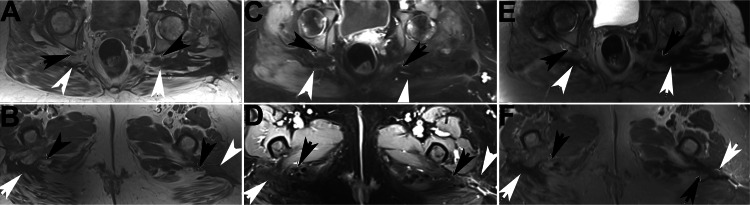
Sequential axial MR images of Case 1 Left column (A, B): T1 sequence; middle column (C, D): T1 fat saturation sequence post contrast; right column (E, F): T2 fat saturation sequence. Black arrows indicate sciatic nerve; white arrows indicate tumor
